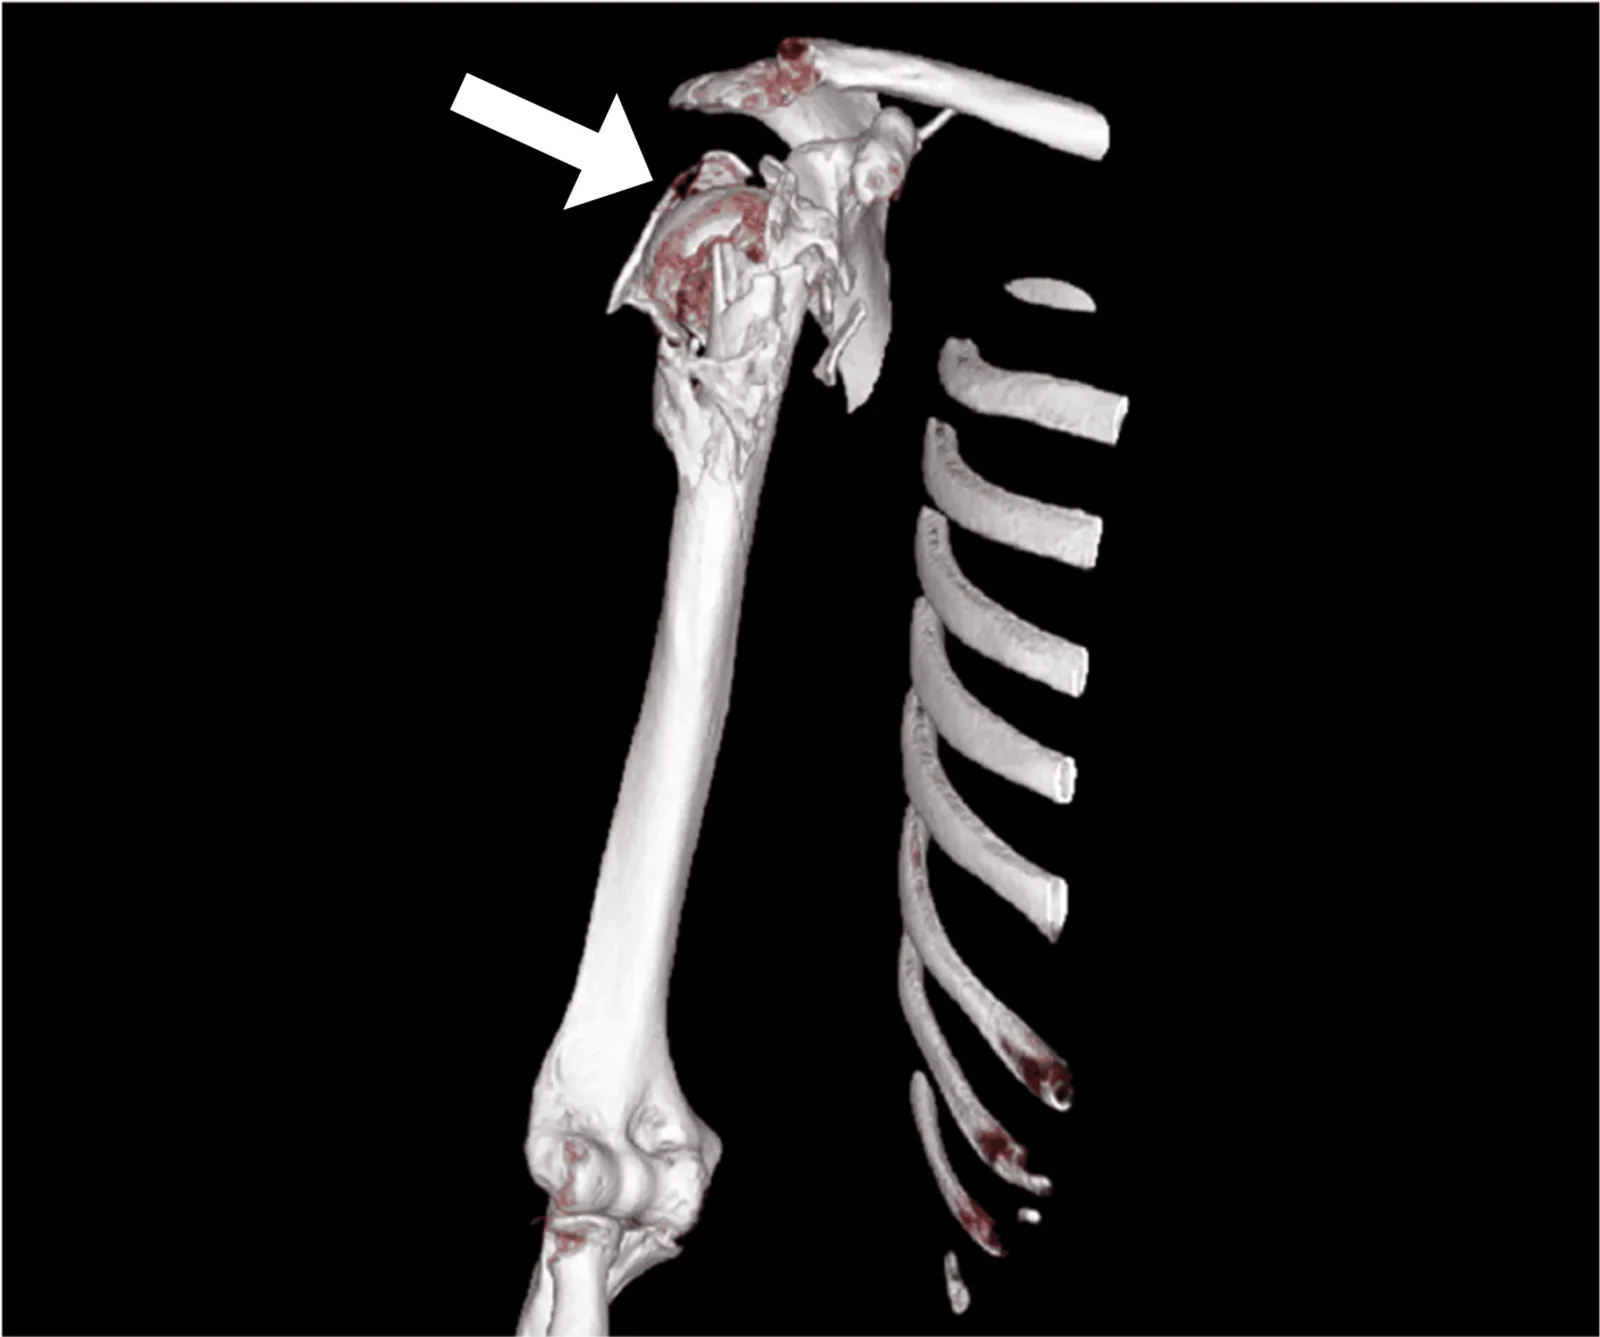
Three-dimensional visual of the patient’s right proximal humerus from the culmination of multiple views on CT demonstrating severe comminution and malunion/nonunion White arrow points to the comminuted fracture of the proximal humerus.
